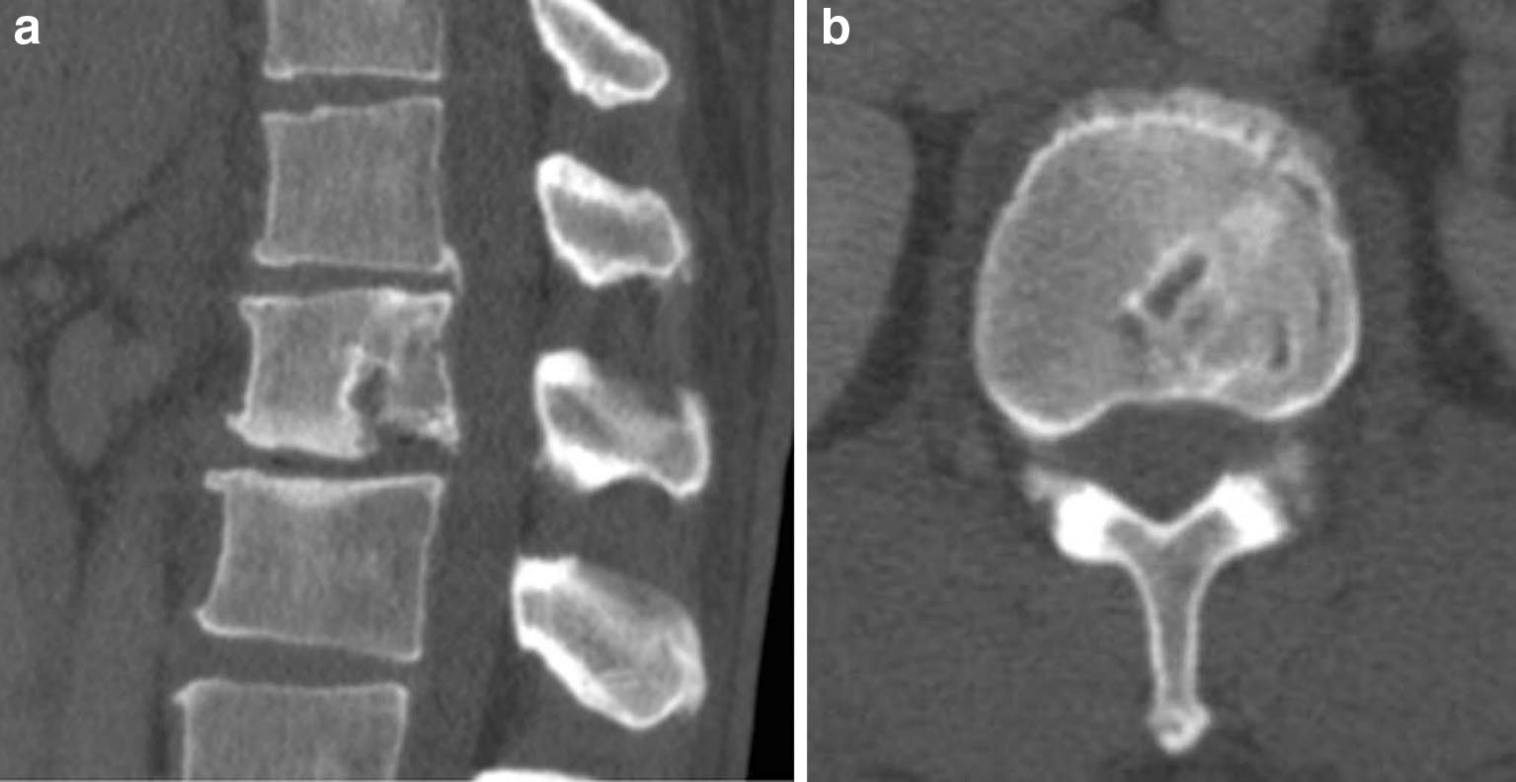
Sagittal (a) and axial (b) computed tomography a year after biopsy showing bone remodeling of the previous osteolytic lesion of the L1 vertebral body
